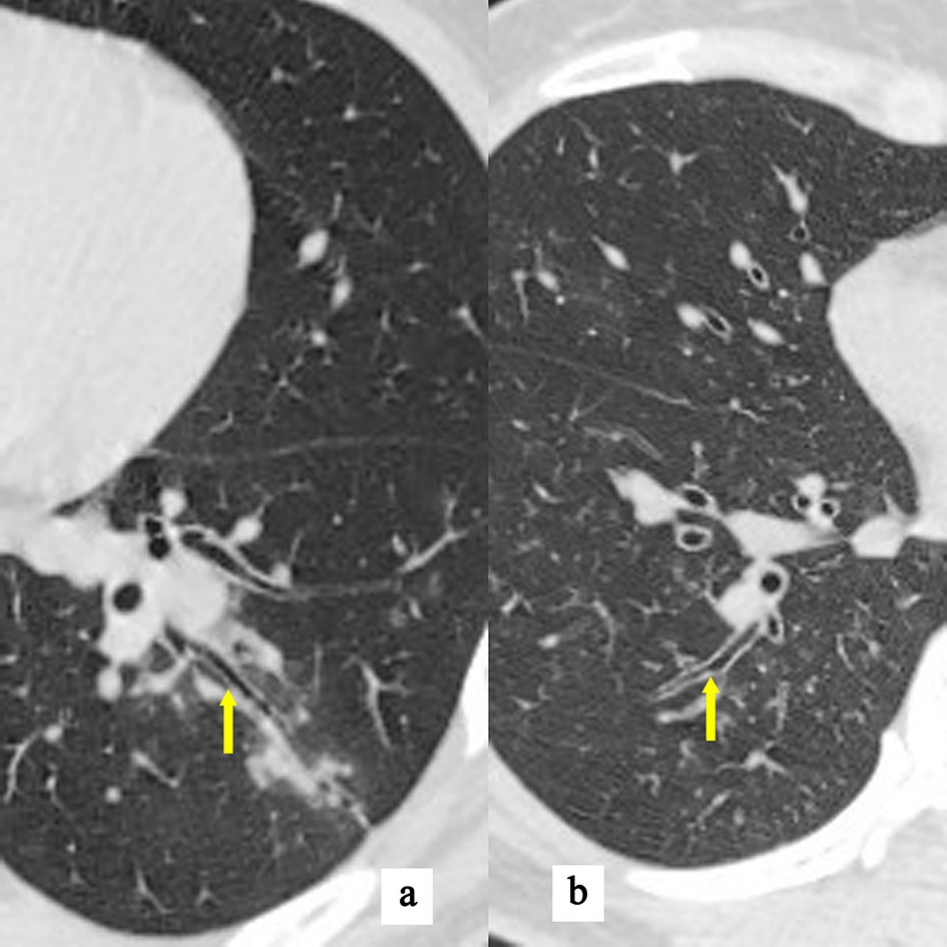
HRCT findings of a 41-year-old male case with mycoplasma pneumoniae pneumonia. The yellow arrow showed the bronchial wall thickening.
